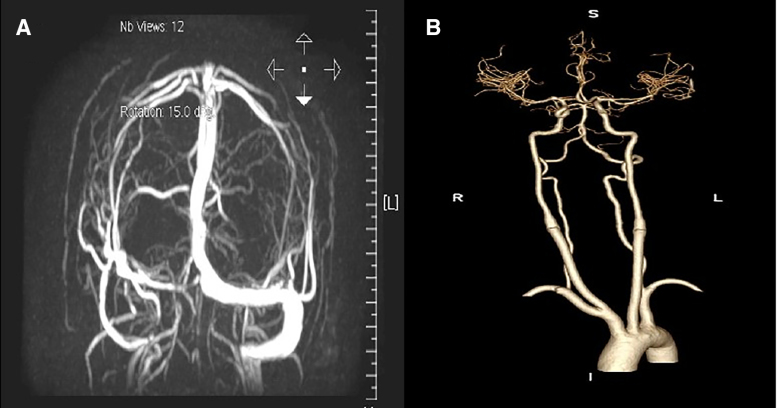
(A) Cranial magnetic resonance venography: The right transverse sinus and sigmoid sinus are relatively slender, suggesting left-sided dominance; (B) Computed tomography angiography of the head and neck: The cerebral vessels showed no significant stenosis.
